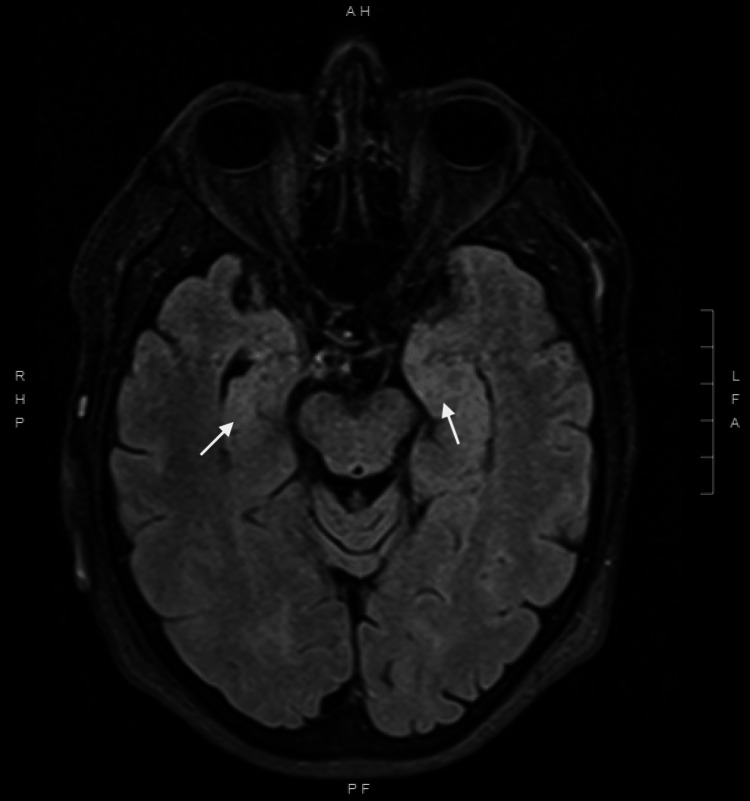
MRI brain showing mild hyperintensities isolated to the bilateral temporal lobes (arrows). These findings are consistent with limbic encephalitis, and more diffuse hyperintensities outside of the temporal lobes would be expected in viral encephalitis MRI: magnetic resonance imaging
